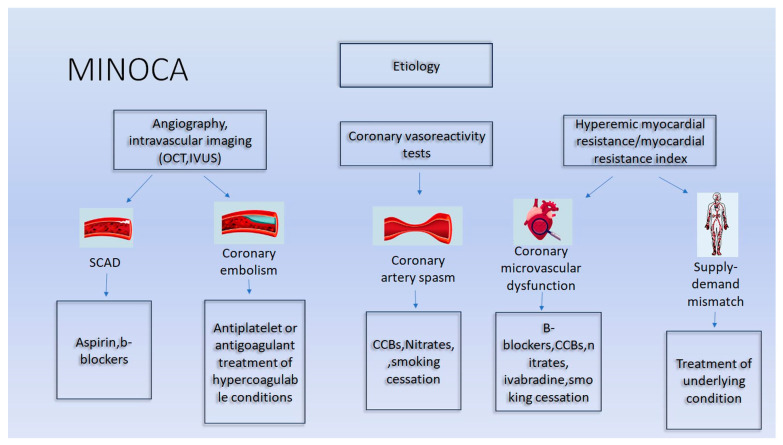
Therapeutic algorithm of MINOCA based on the underlying causes (atherosclerotic and non-atherosclerotic). CCB, calcium channel blockers; IVUS, intravascular ultrasound; oct, optical coherence tomography.
